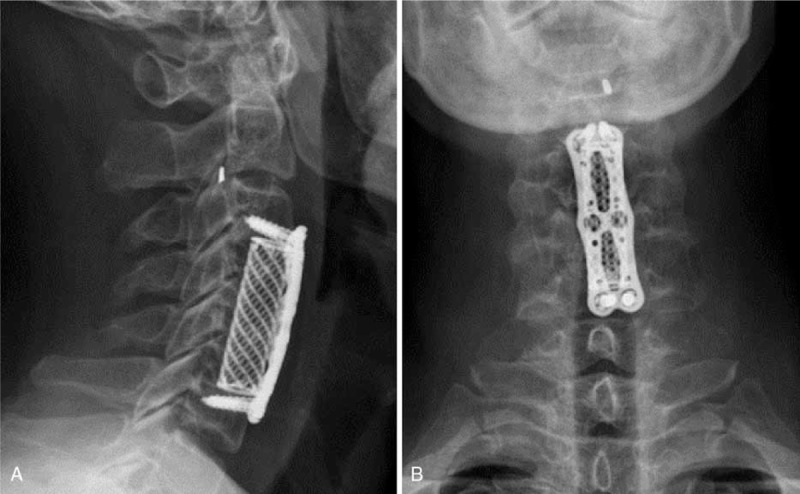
Postoperative x-ray showed the metal fragment was located in the canal immediately behind C2/3 disc (A) and close to left foramen (B).
